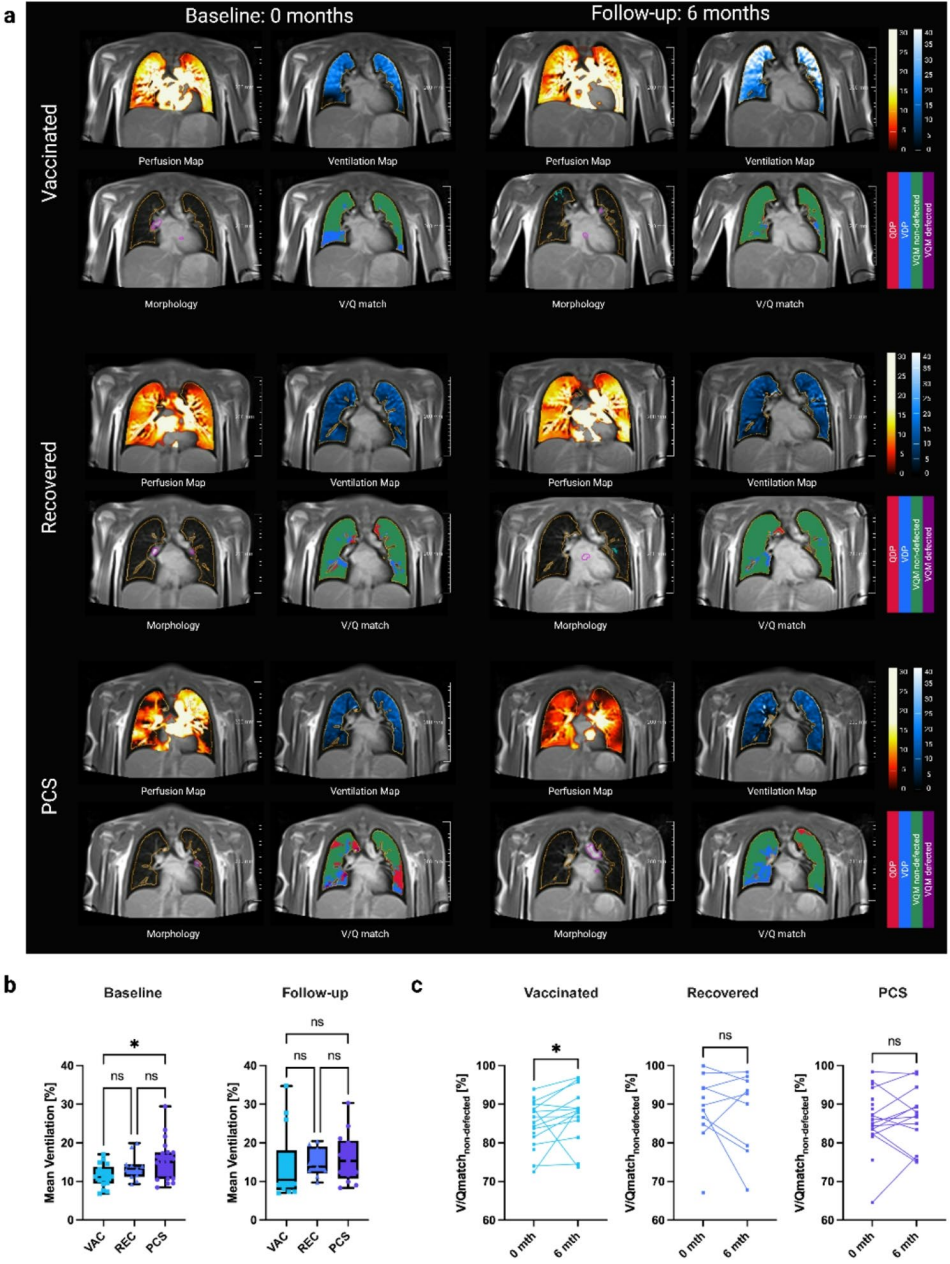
Representative PREFUL MRI scans at baseline and 6-month follow-up. a Representative PREFUL MRI scans of a vaccinated, recovered and Post-COVID-19 syndrome participants at baseline and 6-month follow-up. For each timepoint a perfusion, a ventilation map, morphology and ventilation/perfusion match are displayed. Provided scales are 200mm. b Comparisons of vaccinated, recovered patients, and PCS patients. Vaccinated showed lower mean ventilation than PCS, at follow-up ventilation of vaccinated had increased and no difference remained between cohorts. P values were assessed with Kruskal-Wallis test. c From baseline to follow-up vaccinated showed increased V/Qmatchnon-defected. P values were assessed with Wilcoxon matched-pairs signed rank test. Vac = Vaccinated, Rec = Recovered, PCS = Post-COVID-19 syndrome, PREFUL MRI = Phase-resolved functional lung low-field magnetic resonance imaging, V/Qmatchnon-defected = ventilation perfusion match of non-defected areas. Asterisks represent significant differences. *P ≤ 0.05
